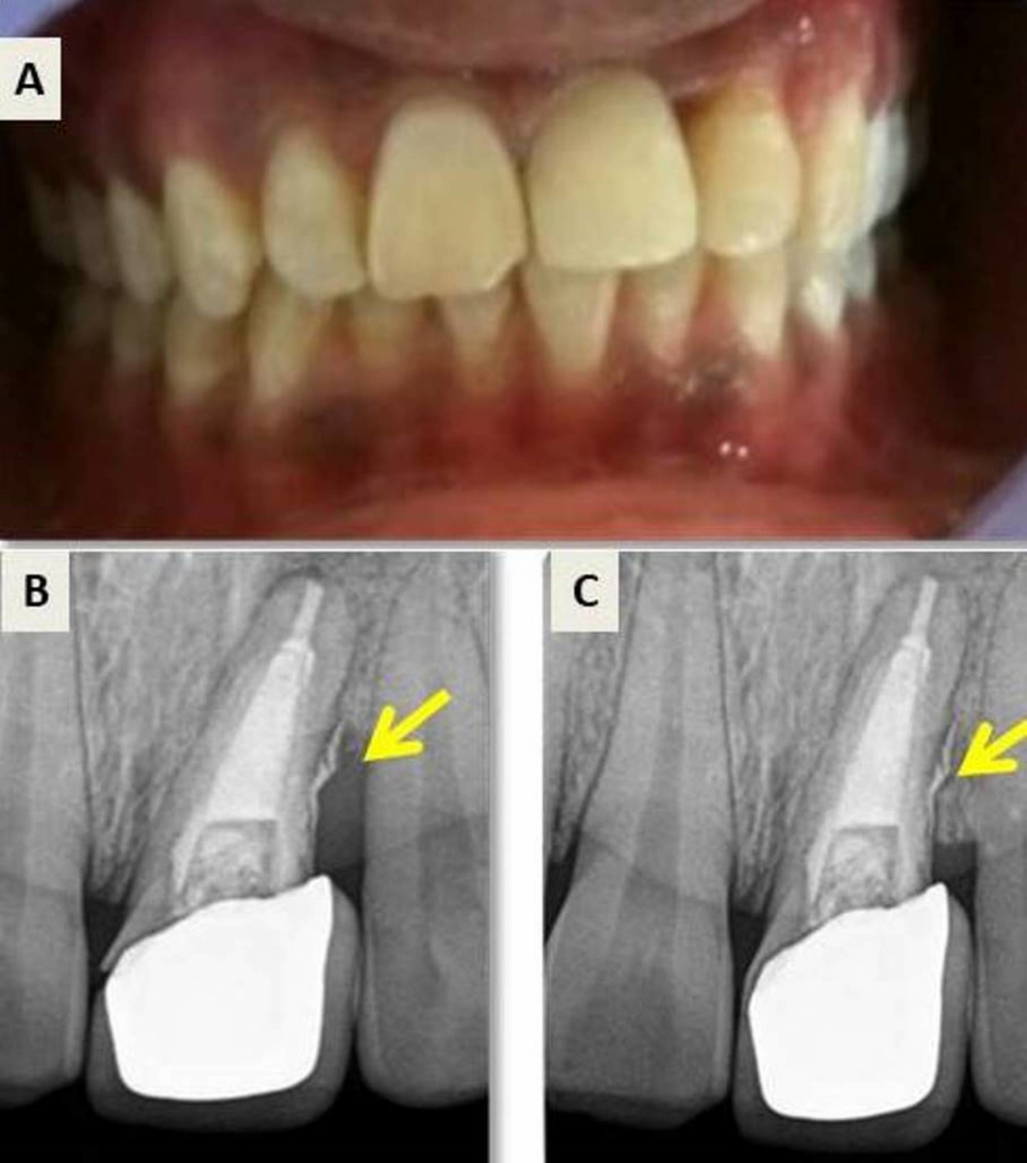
Postoperative photograph and follow-up radiograph. (A) Post-operative photograph of the patient. (B) Six-month follow-up. (C) 18 months follow-up showing healing with bone formation.
